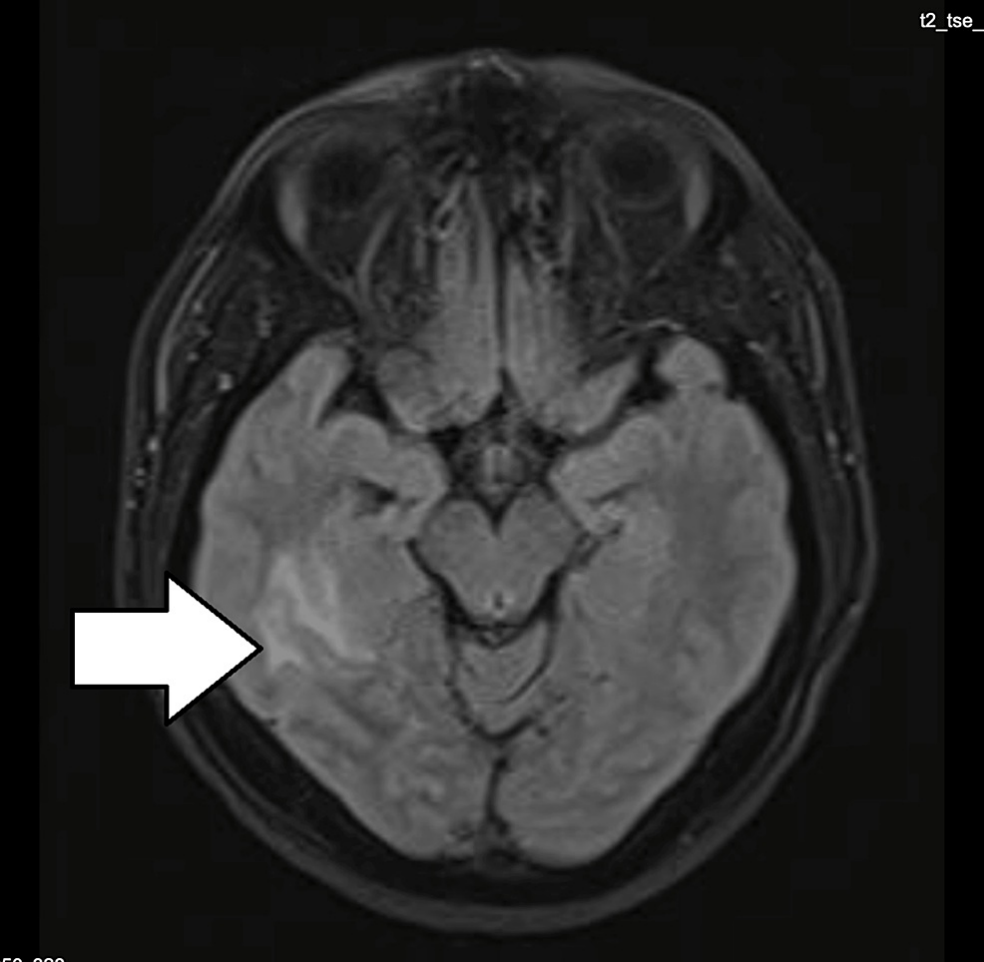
MRI of the brain: T2 flair showing temporal lobe hyperintensity in the right temporal lobe
